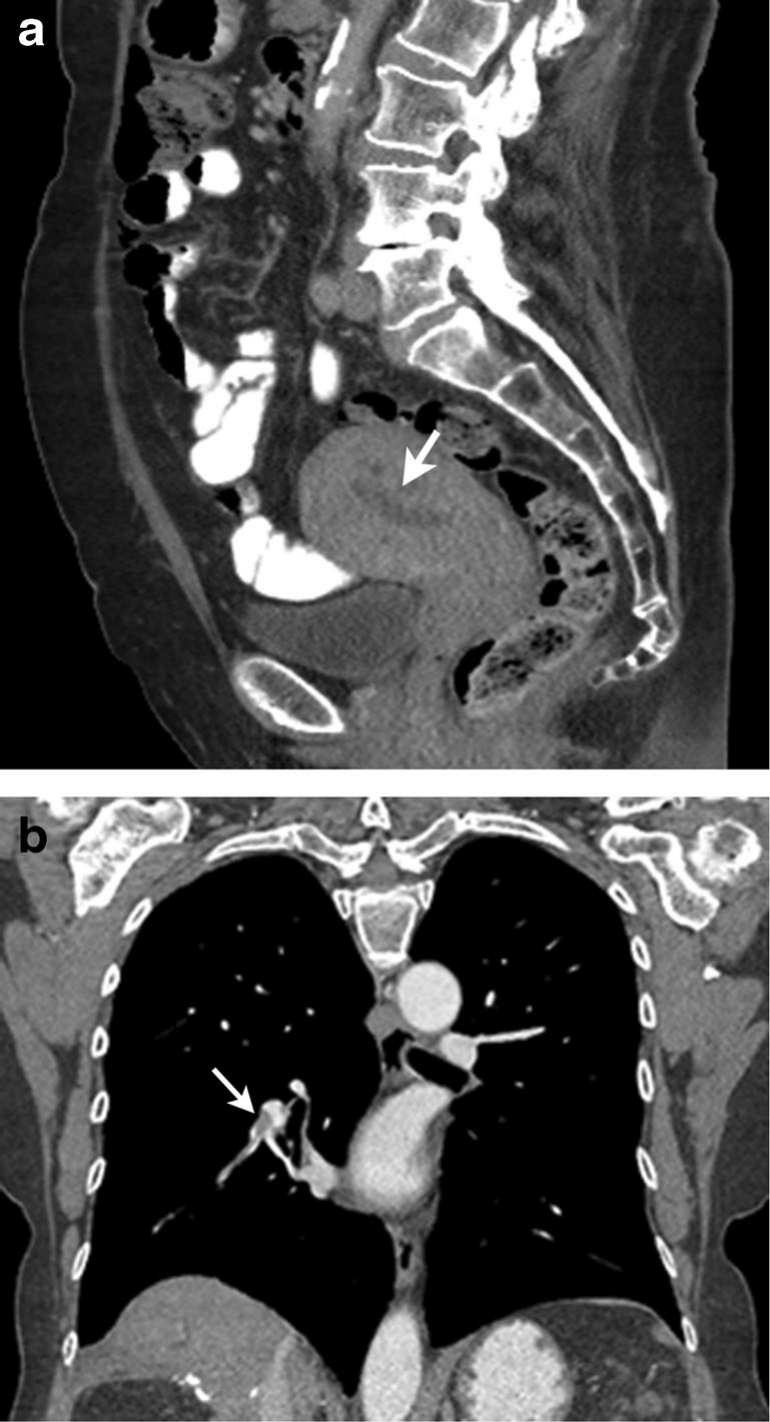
72-year-old woman with metastatic hormone receptor-positive breast cancer being treated with tamoxifen. a Sagittal contrast-enhanced CT image of the pelvis shows irregular thickening of the endometrial lining (*arrow*) suggestive of endometrial hyperplasia. b Coronal contrast-enhanced CT image of the pelvis during routine restaging study shows hypodense filling defect (*arrow*) in descending branch of right pulmonary artery suggestive of pulmonary embolism. The patient had no complaints of chest pain
